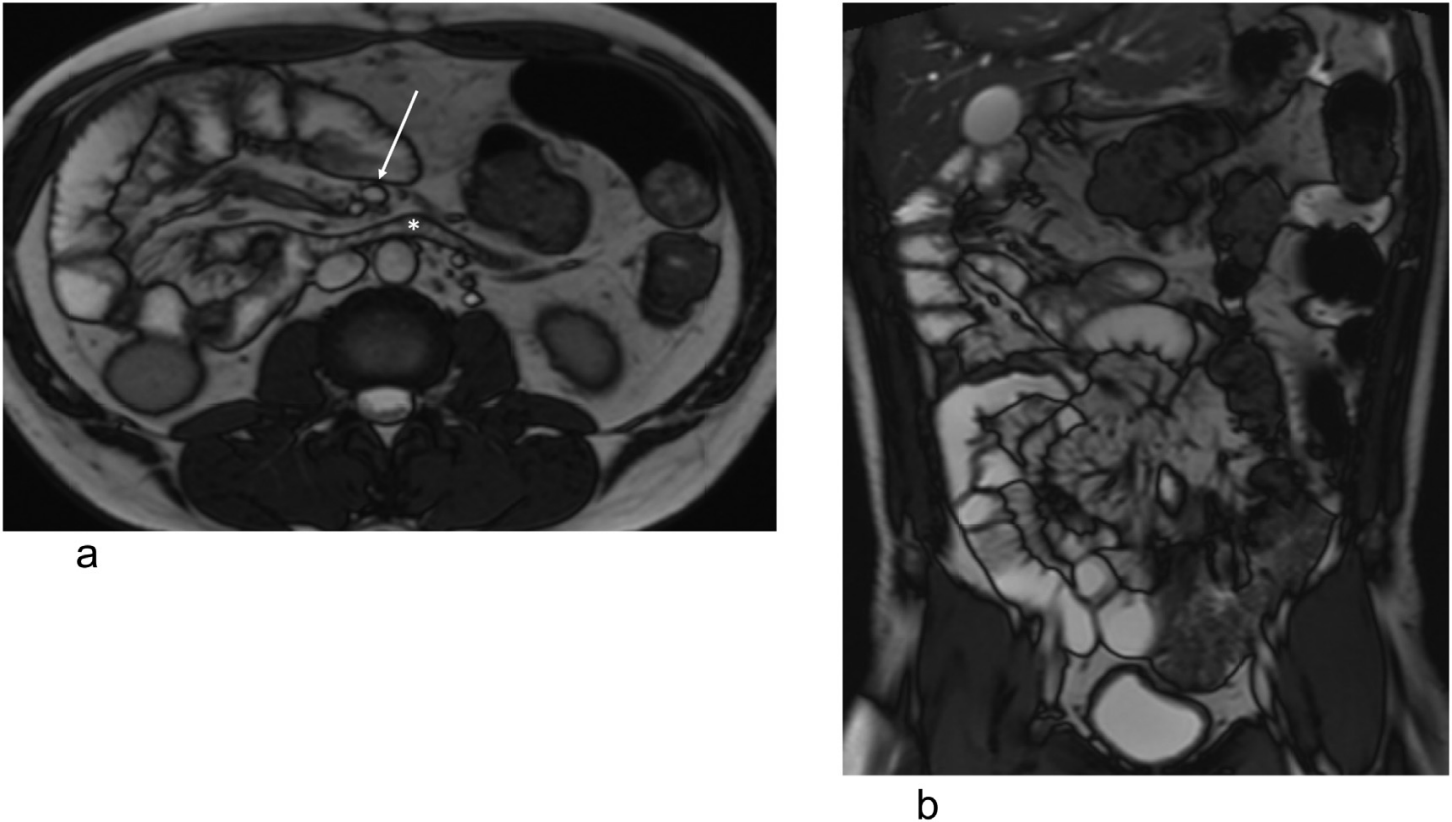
a- MRI, axial ‘True FISP’ image showing congenital small bowel malrotation with reversed orientation of the superior mesenteric vessels (arrow). Although the 3rd part of the duodenum (*) is normally sited, the DJ flexure and small bowel loops lie to the right of the midline with the colon seen on the left. b- MRI, coronal ‘True FISP’ image showing congenital small bowel malrotation with small bowel loops in the right side of the abdomen and colon on the left.
